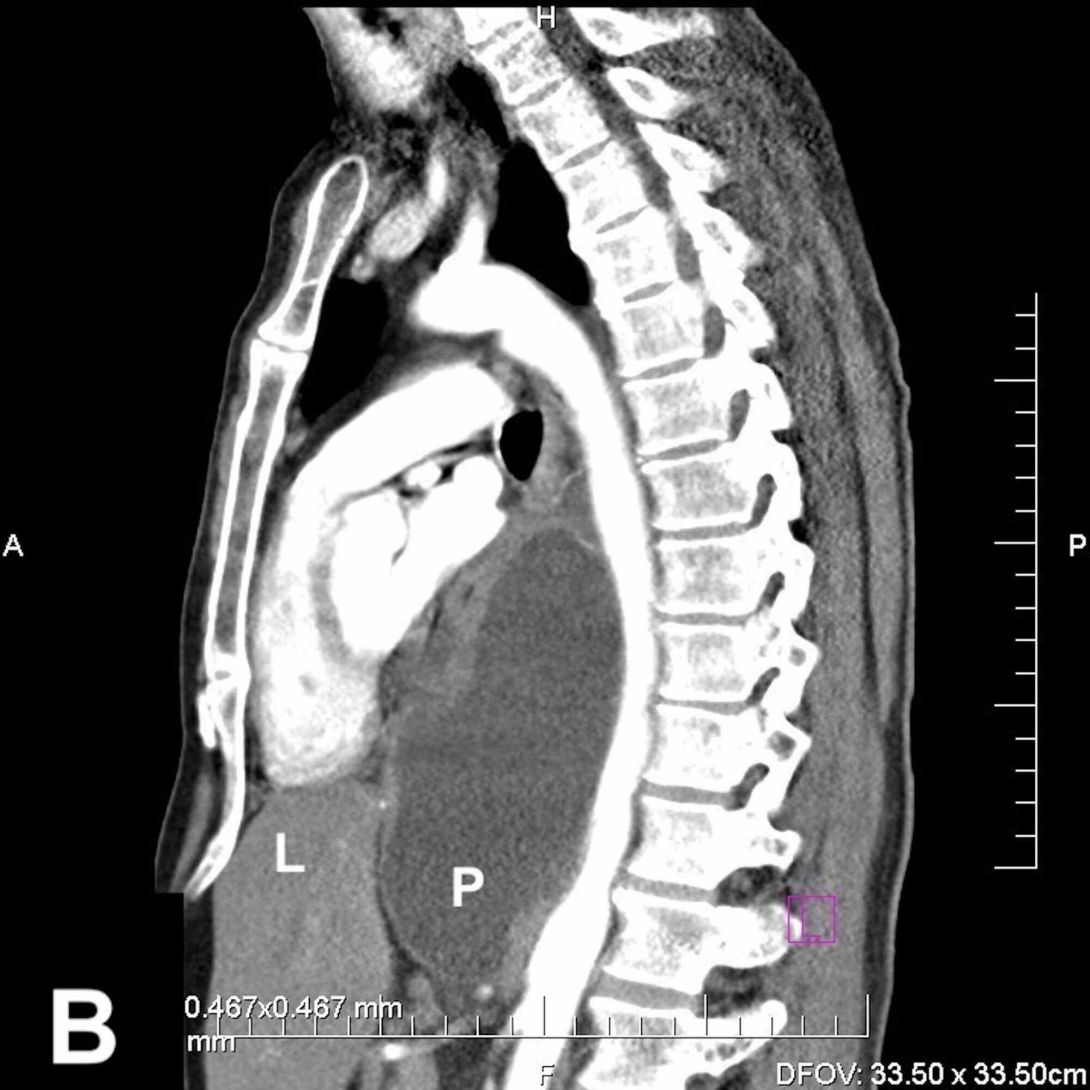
Chest CT scan (sagittal) demonstrate a longitudinal fluid chollection (P-pseudocyst) in the mediastinum, along the thoracic aorta. (L-liver)
